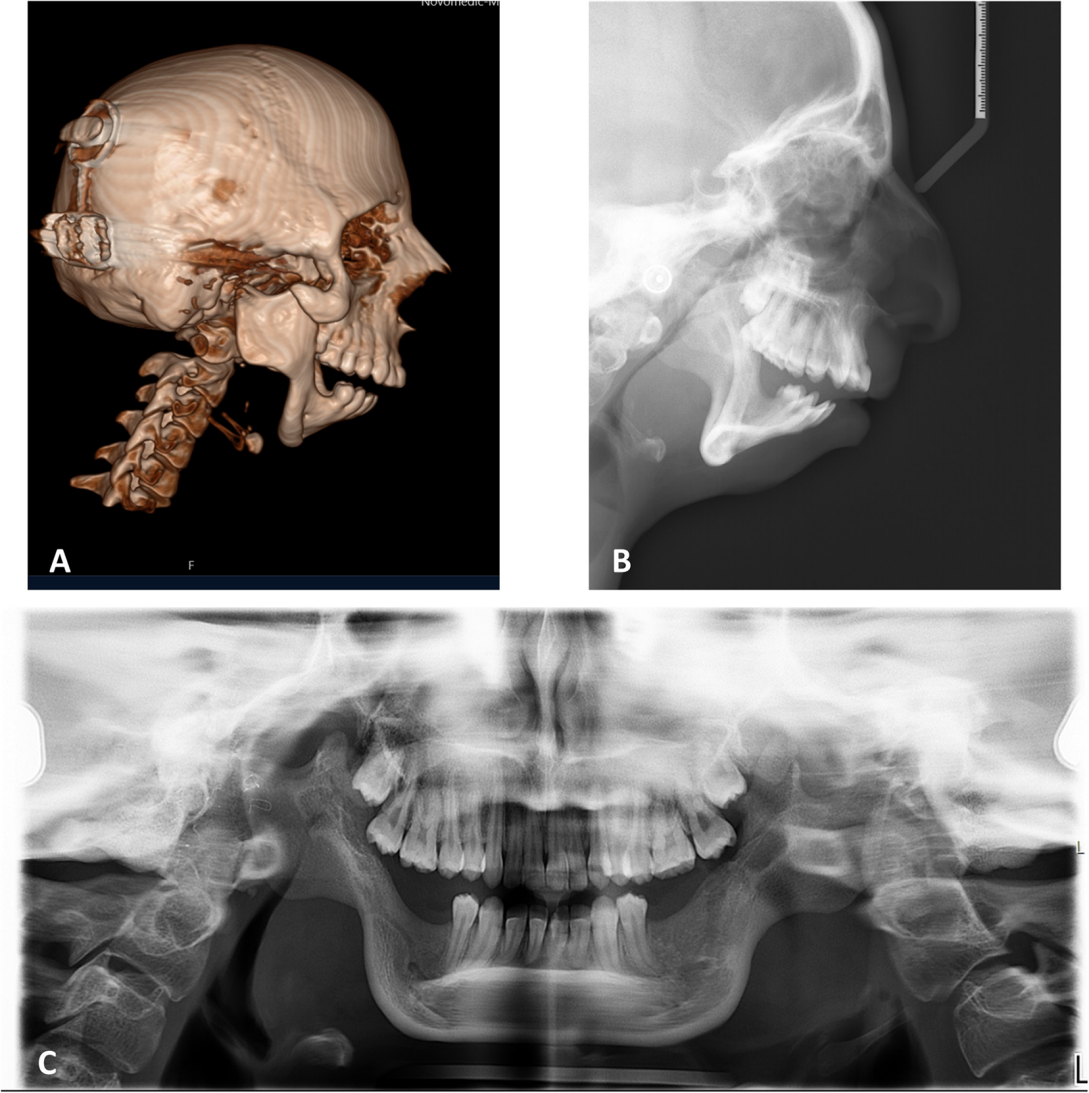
Preoperative radiography. (A) Sagittal view of CBCT and lateral cephalometry (B) demonstrate the retruded position of the pogonion, short ramie, missing condyles, deep mandibular antegonial notches, all associated with TCS phenotype. (C) Panoramic radiograph emphasizing the symmetry of the condition.
